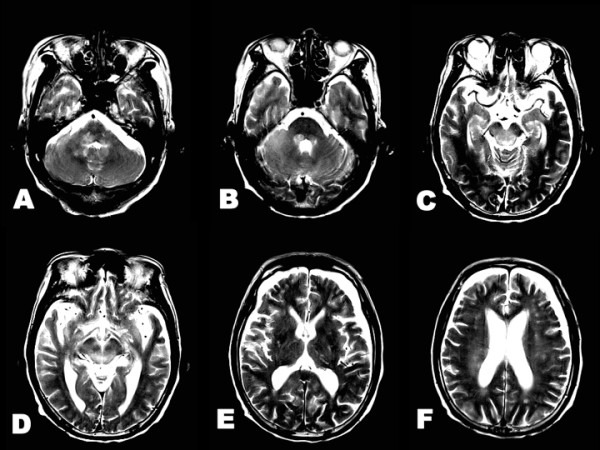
Brain T2-weighted imaging at neurological onset. A-F. Hyperintense lesions are observed in the dorsal pons, midbrain, cerebellum and periventricular white matter. E. There were no remarkable changes in the basal ganglia.
